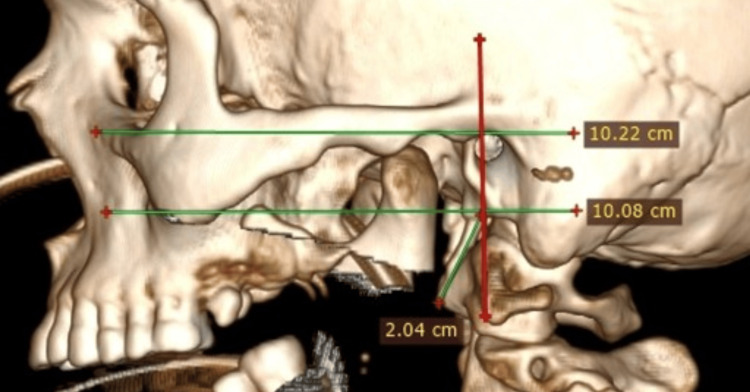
A 3D CT lateral-view image showing the styloid process, a Frankfurt line (upper line), a horizontal line (lower line) drawn at the base of the styloid process, and a vertical line crossing the horizontal line at the centre of the base of the styloid process. 3D CT, three-dimensional computed tomography.
